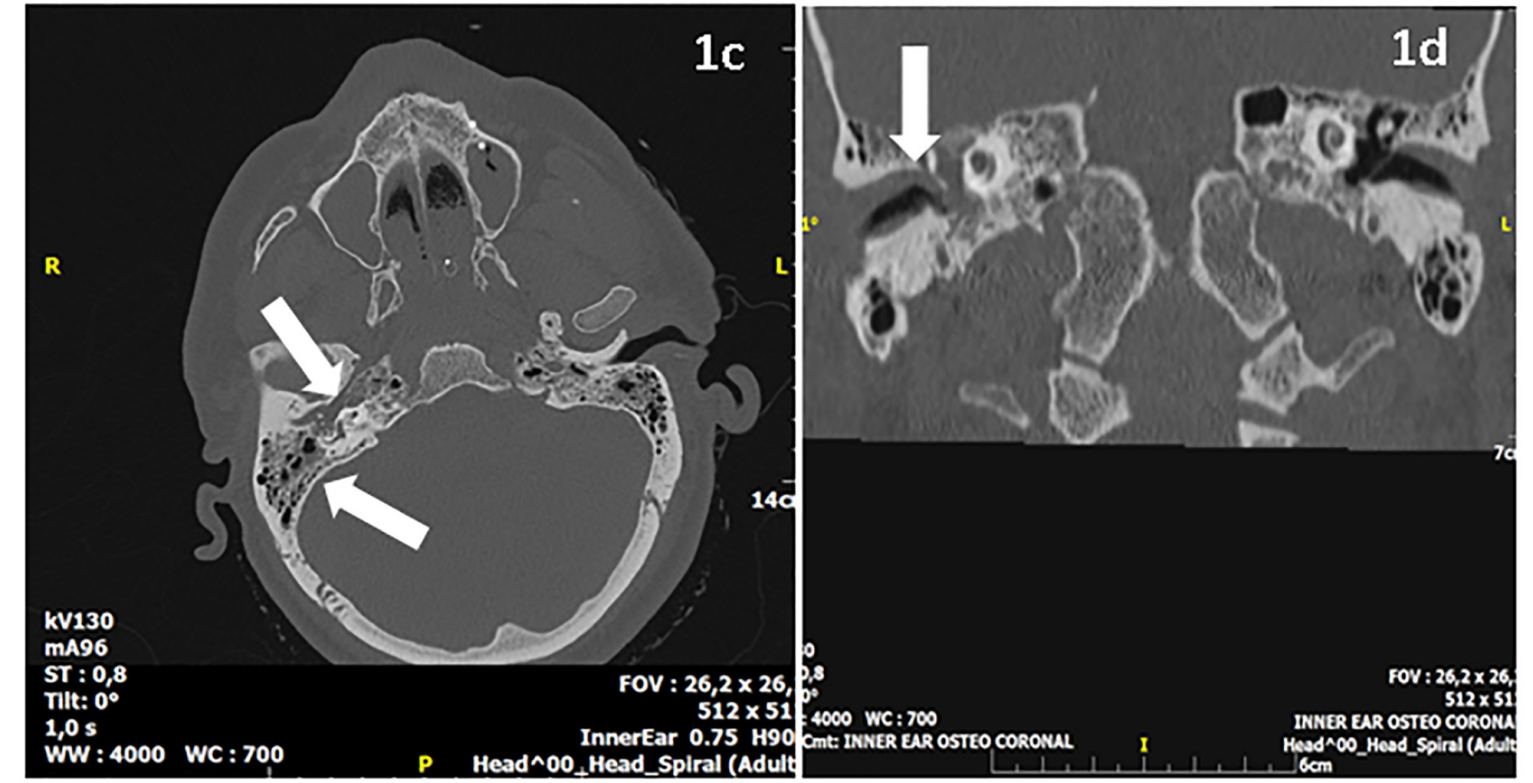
Axial brain and inner ear osteo-coronal CT images Axial brain and inner ear osteo-coronal CT images showing otitis media and mastoiditis on the right side. Presence of soft tissue density in the right middle ear cavity and the mastoid with hypo-pneumatization. CT: computed tomography
